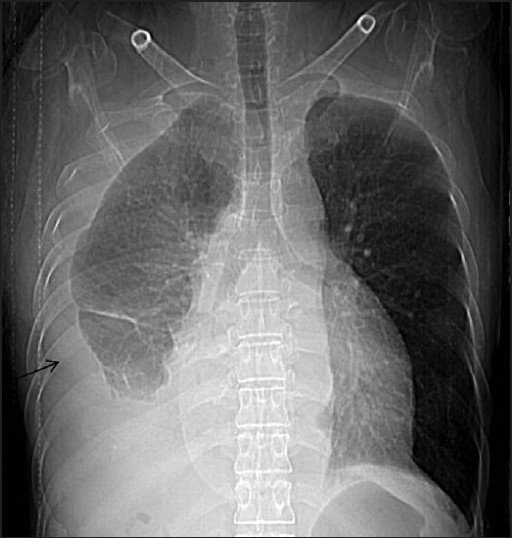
Computed tomography scanogram showing right pleural effusion
